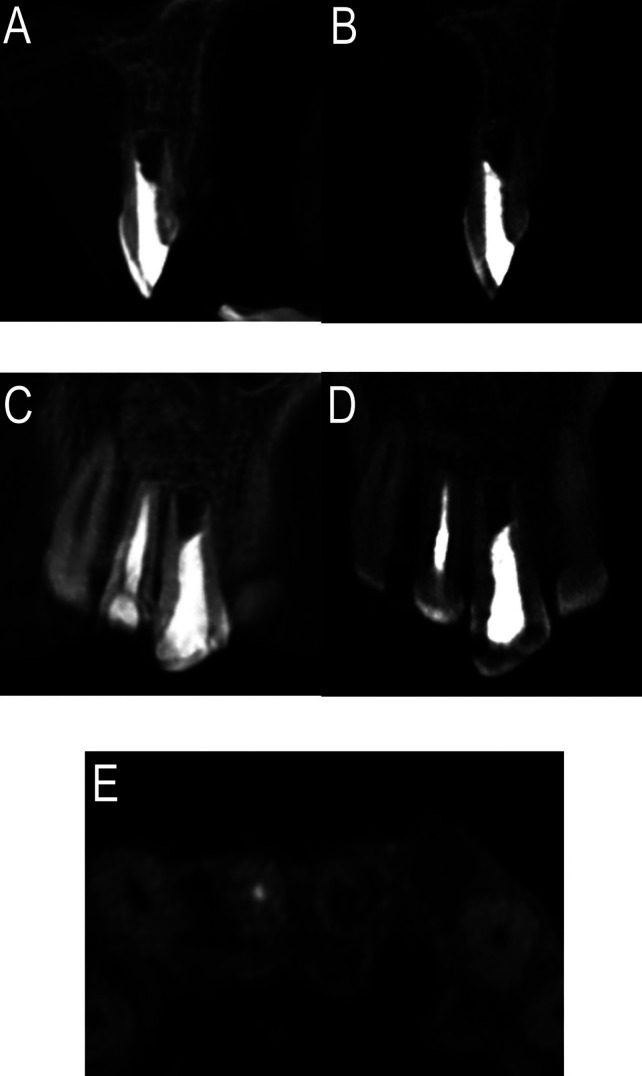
Images of cone-beam computed tomographic scan visualized by Planmeca Romexis Viewer v. 3.4.1.R. at two years (A and C) and Kodak Dental Imaging Software 3D Module v 2.4 at six years (B, D and E). A and B) Sagital slice at 1.1 level at two and six years. C and D) Coronal slice at two and six years. E) Axial slice at six years. All of them show the complete resolution of the extensive apical lesion and bone regeneration, with a slight ingrowth of bone-like tissue inside of the root canal space in the apical portion of the tooth and a slightly increase of root length at 6-years follow-up.
